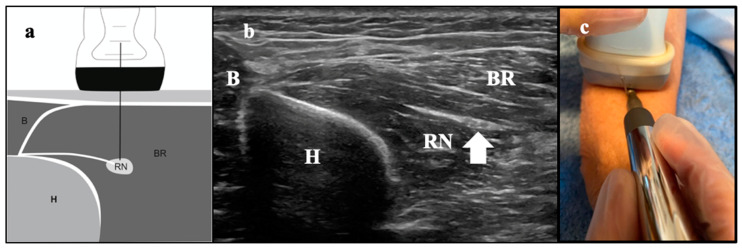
US-Guided PNM: (a) PES intervention in the radial nerve; (b) Ultrasound image of the intervention. B: brachialis muscle; BR: brachioradialis muscle, RN: radial nerve, H: humerus; and (c) ultrasound-guided invasive approach of the radial nerve.
